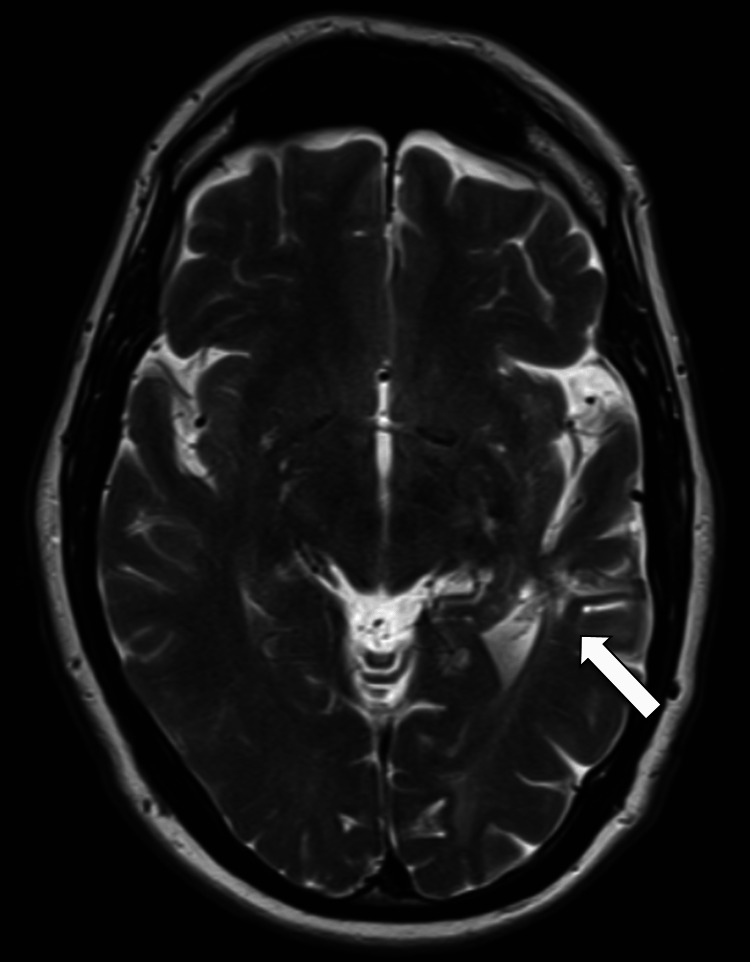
Magnetic resonance imaging of the head (T2W post-contrast) depicting the area of encephalomalacia of the left temporal lobe (arrow) – previous cerebral abscess.
